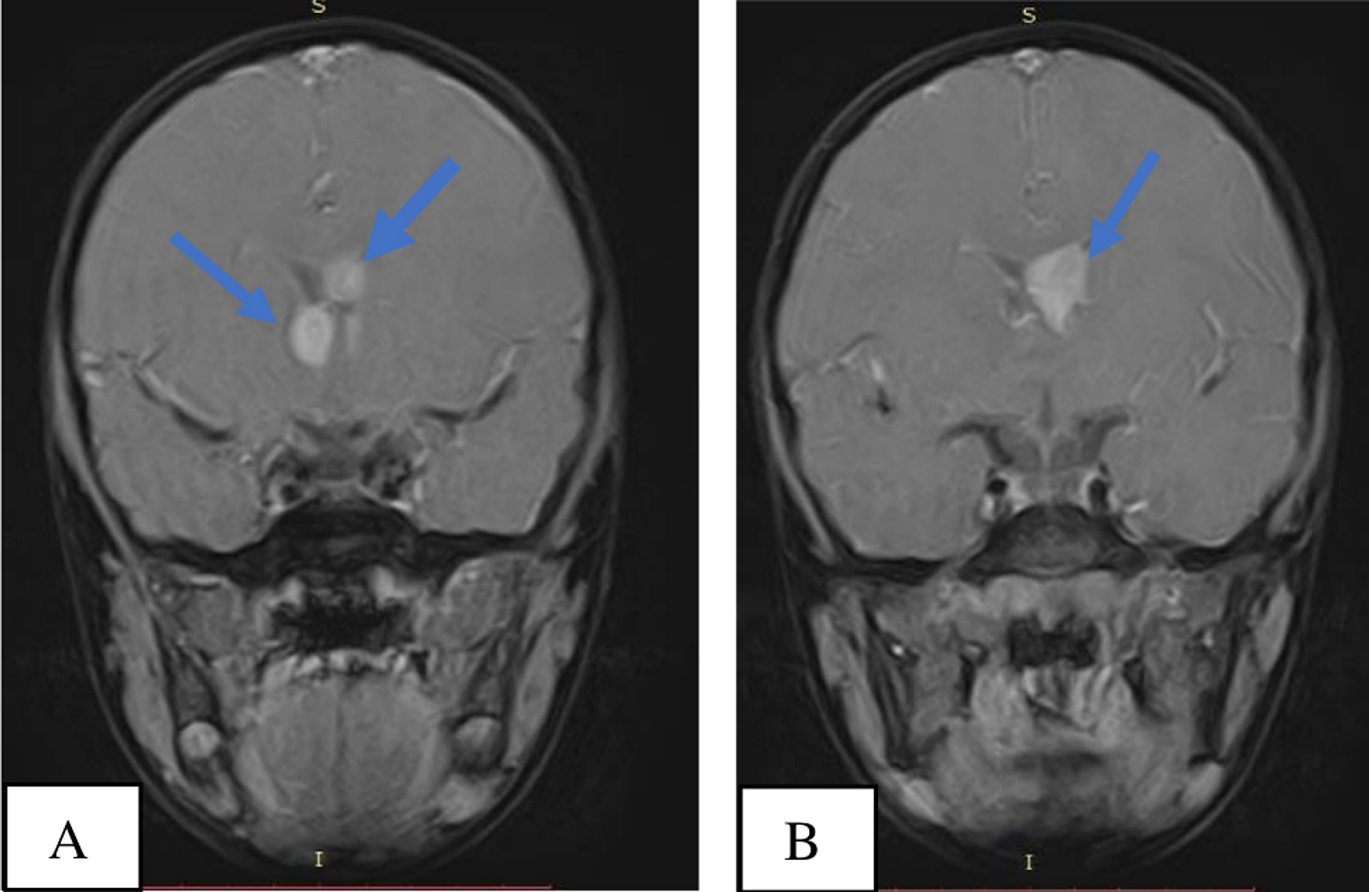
MRI , T1 Coronal FatSat contrast enhanced images showing the right & left Sub ependymal Giant Cell Astrocytomas (blue arrows) (5A, 5B) approaching the Foramina of Monroe . No resultant hydrocephalus.
